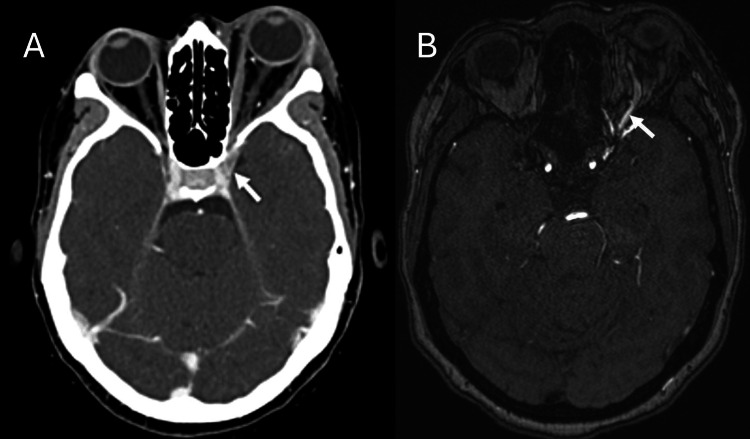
(A) Axial contrast-enhanced CT of the brain showing partial opacification and engorgement of the left cavernous sinus (arrow); (B) axial MRI angiography demonstrating early enhancement of the superior ophthalmic vein (arrow) in the arterial phase.
